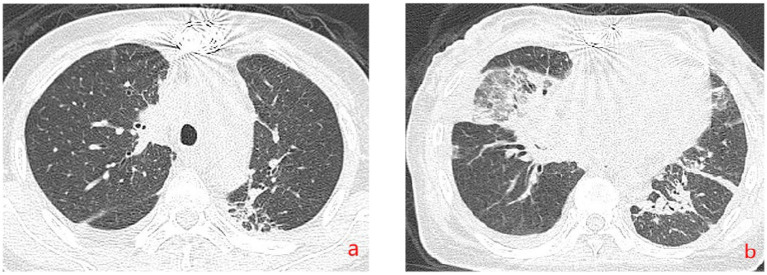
Chest CT (December 29, 2024). (a,b) demonstrate post-pericardial stripping changes. Mediastinal exudate and effusion are decreased compared to previous findings. Uneven thickening of the pericardium and mediastinal soft tissues is reduced. Lesions in the right lung are increased compared to previous findings.
